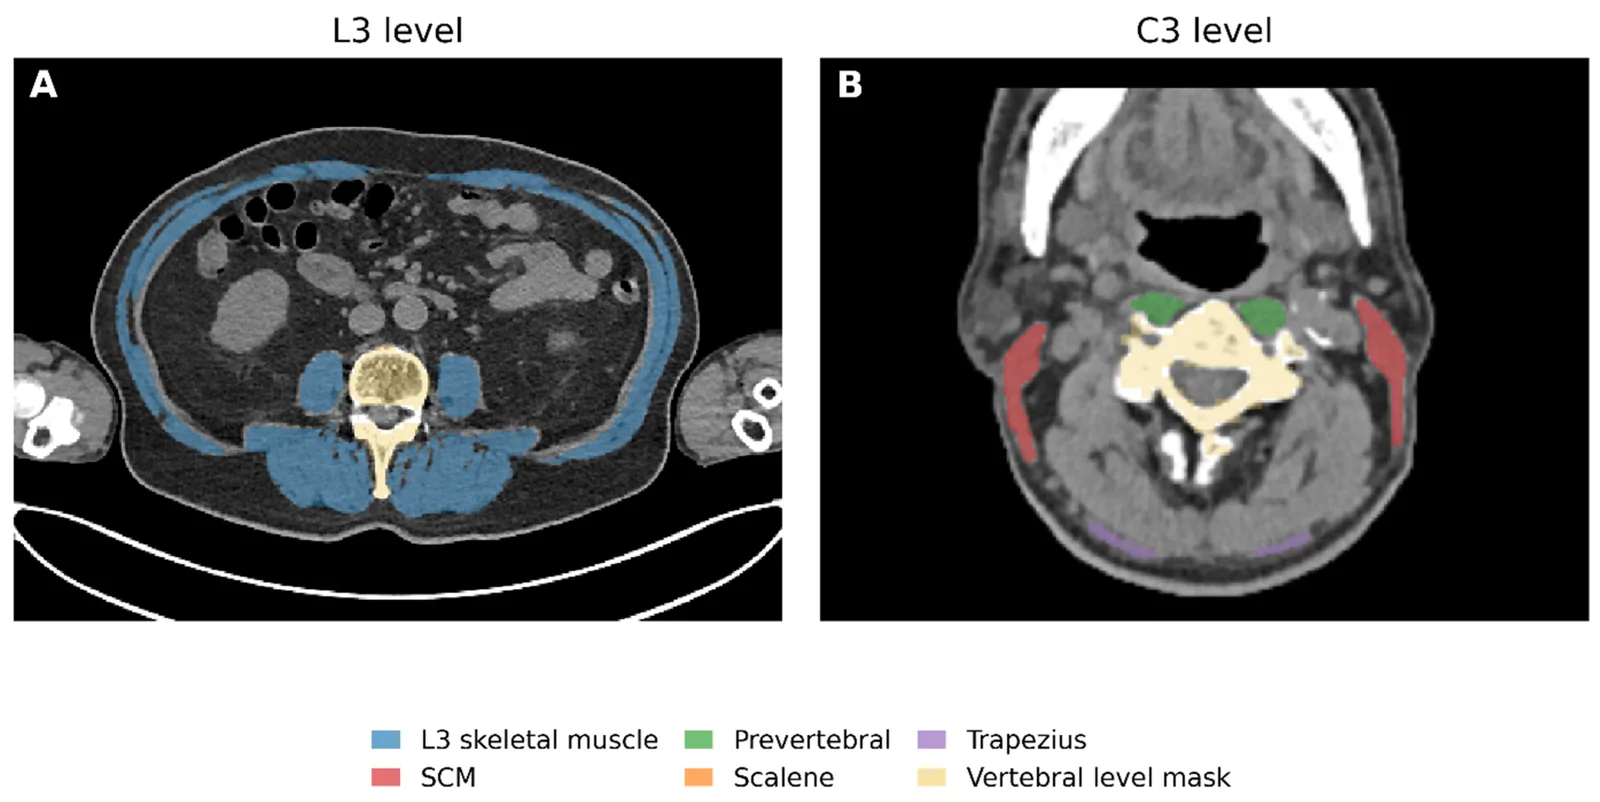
Representative examples of CT segmentation. Axial CT images from one representative case showing the segmented regions used for body composition measurements. (A) At the L3 level, the skeletal muscle within the torso was segmented to calculate the L3 skeletal muscle index. (B) At the C3 level, the sternocleidomastoid, prevertebral, scalene, and trapezius muscles were segmented to calculate the C3 head-and-neck muscle index. The vertebral level mask is indicated in yellow.
